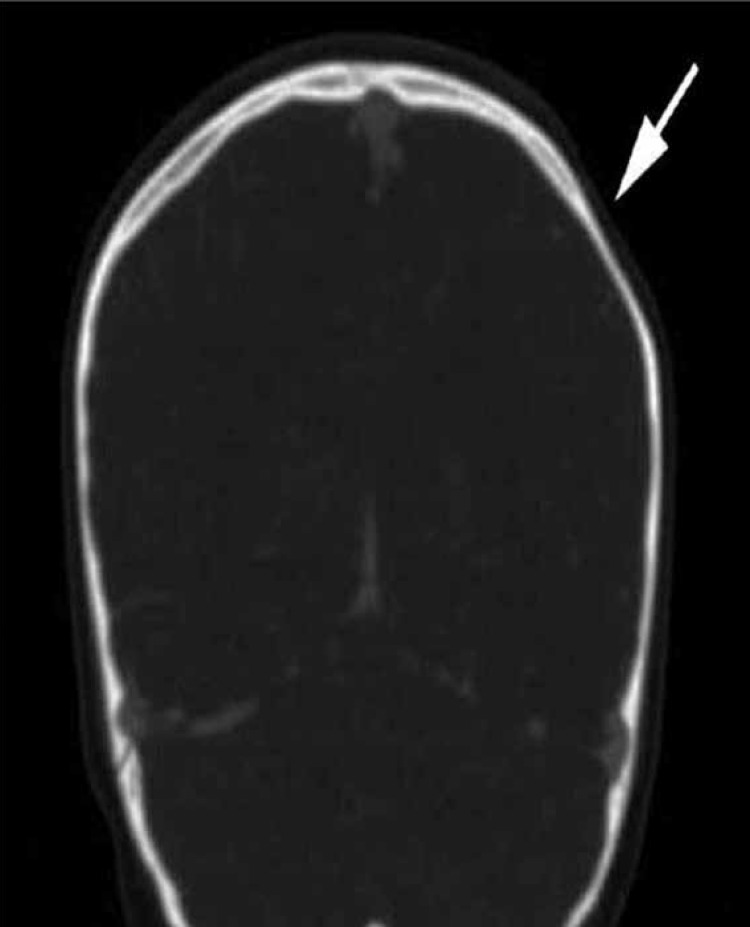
Coronal 2-D reformatted CT image with contrast demonstrates focal scalp thinning within the left fronto-parietal region and thinning of the underlying frontal and parietal bones.
